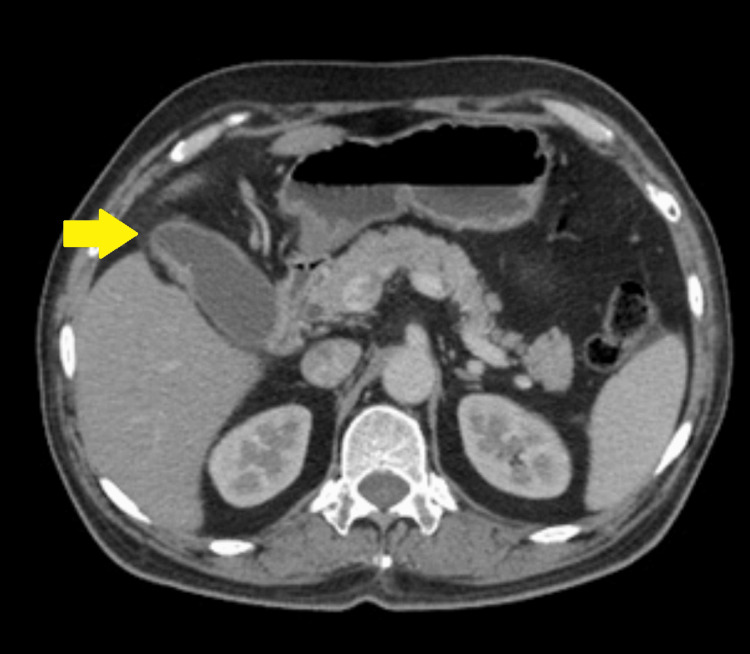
Preoperative contrast-enhanced CT of the abdomen (axial section) showing mild asymmetric mural thickening involving the gallbladder (yellow arrow). Fat planes with the liver are well maintained. Mild pericholecystic fat stranding is present. No enlarged lymph nodes are found.
